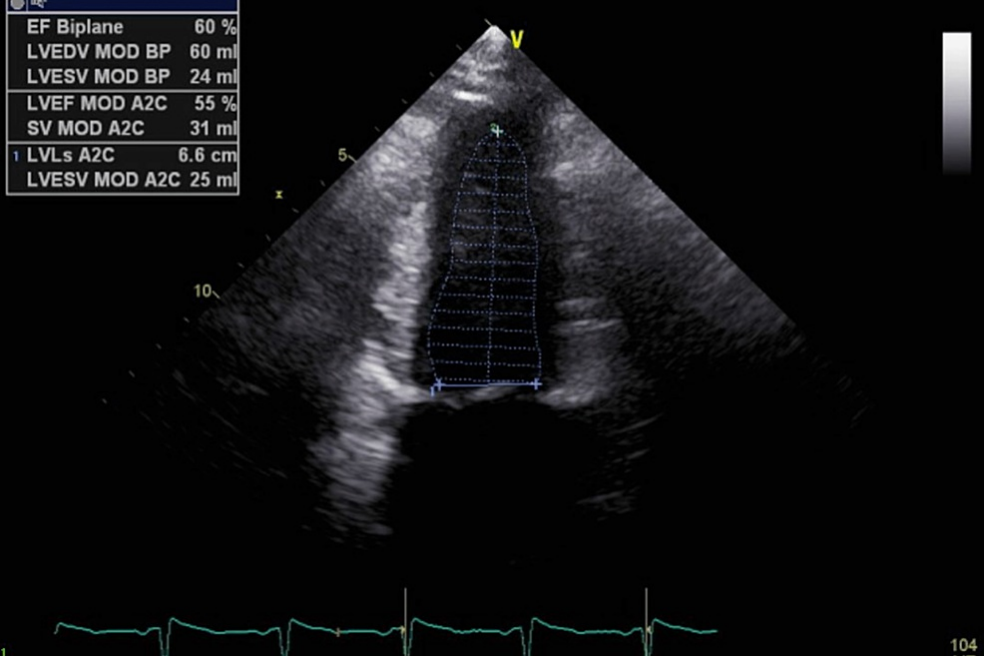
Echocardiogram showing ejection fraction of 55-60% with no evidence of thrombus or atrial or ventricular enlargement
